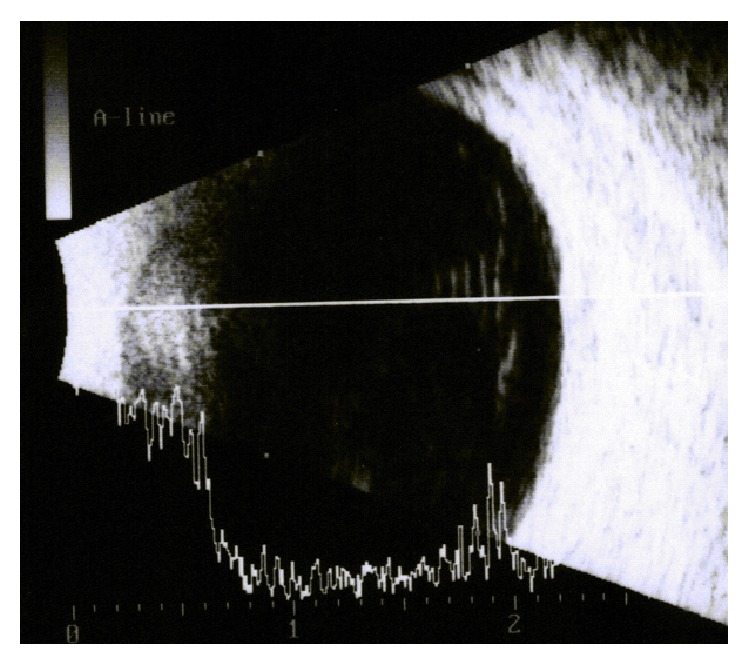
Example of ultrasonography image showing symptomatic PVD in one eye 7 months after multifocal IOL implantation in a patient complaining of floaters, halos, and poor quality of vision.
